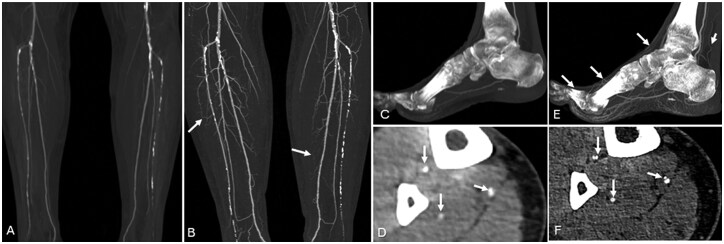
Runoff CT angiography of the lower extremities in 3 different patients. (A) EID-CT 3-dimensional (3D) maximum intensity projection (MIP) showing major lower-limb vessels. (B) PCD-CT 3D MIP from the same patient as (A), demonstrating improved visualization of perforating arteries and small branches. (C, E) 3D MIPs in another patient (C from EID-CT; E from PCD-CT) showing additional fine vessels on the dorsum of the foot not appreciated on the corresponding EID-CT. (D, F) Axial images (D from EID-CT; F from PCD-CT) highlighting small calcified arteries (arrows) with minimal distinction between calcification and lumen on EID-CT but clearly depicted on PCD-CT, with distinct separation of the contrast-filled lumen from calcifications, allowing accurate luminal assessment. Abbreviations: CTA, CT angiography; EID, energy-integrating detector; PCD, photon-counting detector.
